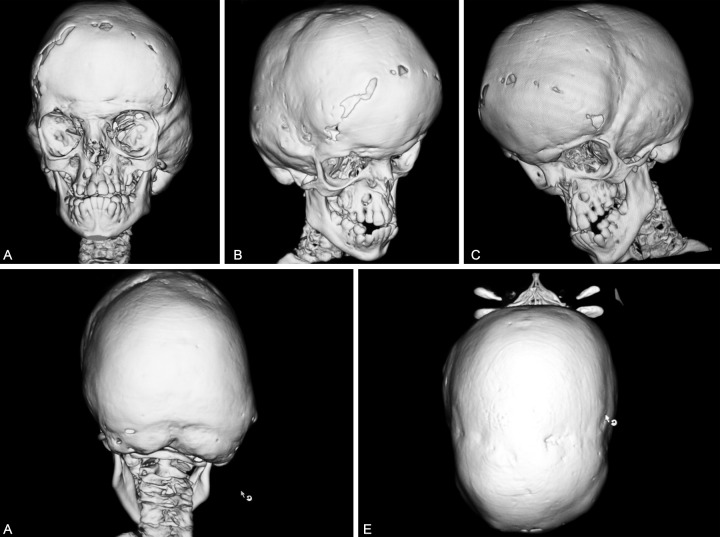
(A to C) Three-dimensional CT scan of skull revealing surgically opened coronal sutures, frontal bossing, shallow orbits, hypoplastic maxilla and zygoma; (D and E) obliteration of sagittal and lambdoid sutures
